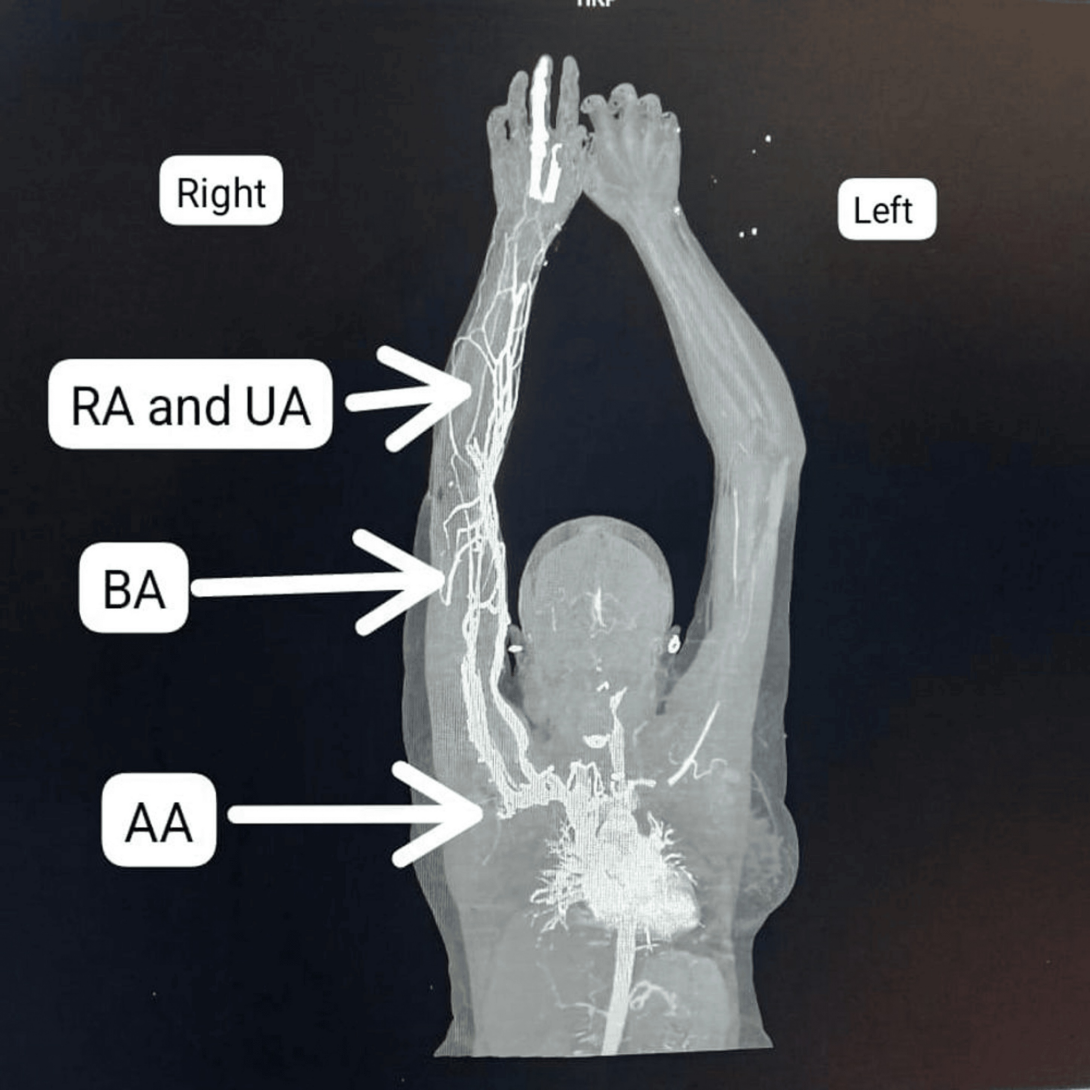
Coronal CT angiographic study of the bilateral upper limbs processed to an anatomical overlay image depicting near-complete thrombosis of the left subclavian and axillary arteries and complete thrombosis of the left radial and ulnar arteries. RA = radial artery; UA = ulnar artery; BA = brachial artery; AA = axillary artery
